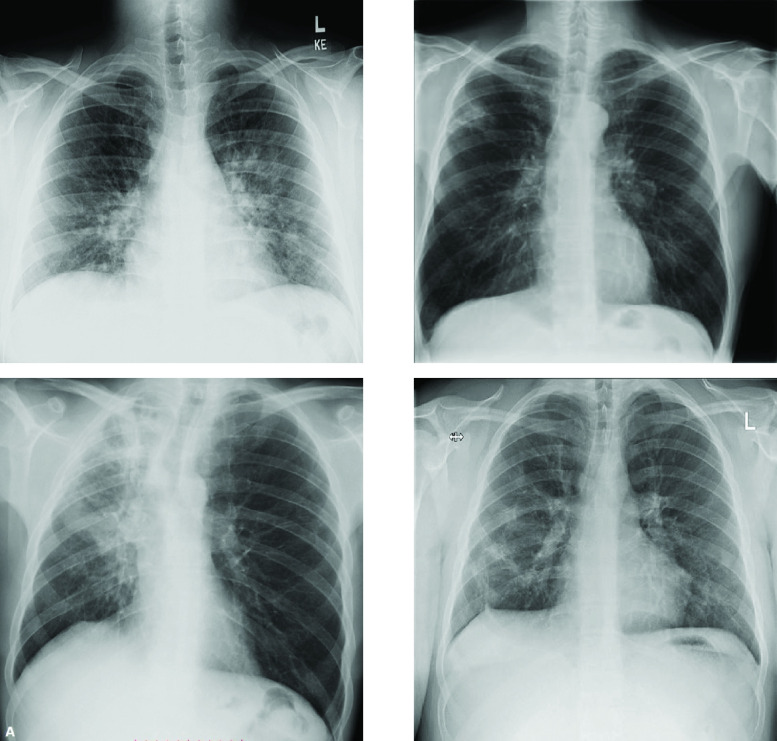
Samples of the original chest X-ray COVID-19 infected cases [31] tested in the experiments.
